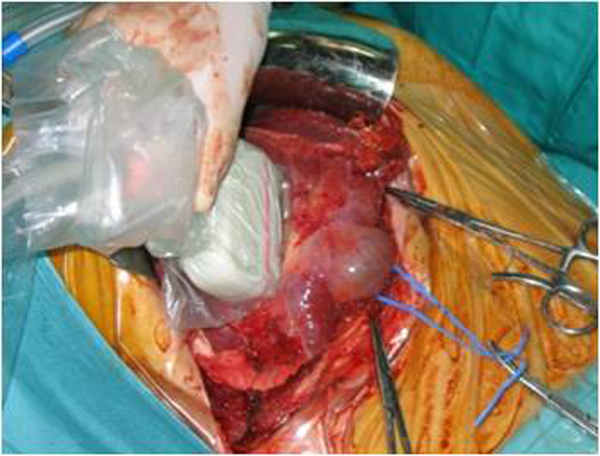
Intraoperatory ultrasound*: the intraoperatory high resolution ultrasound may identify very small liver lesions (including the marginal ones) that are often unrecognized preoperatory with other imaging methods.
